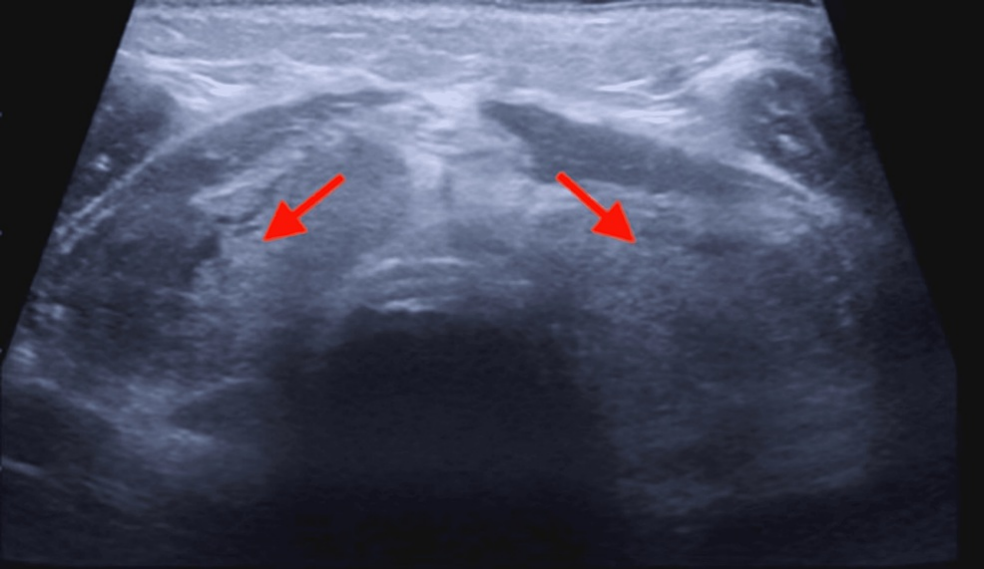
Ultrasound of the thyroid gland, demonstrating heterogeneous enlargement of both lobes of the thyroid gland (red arrows). No hypoechoic lesions or abscess was noted.
